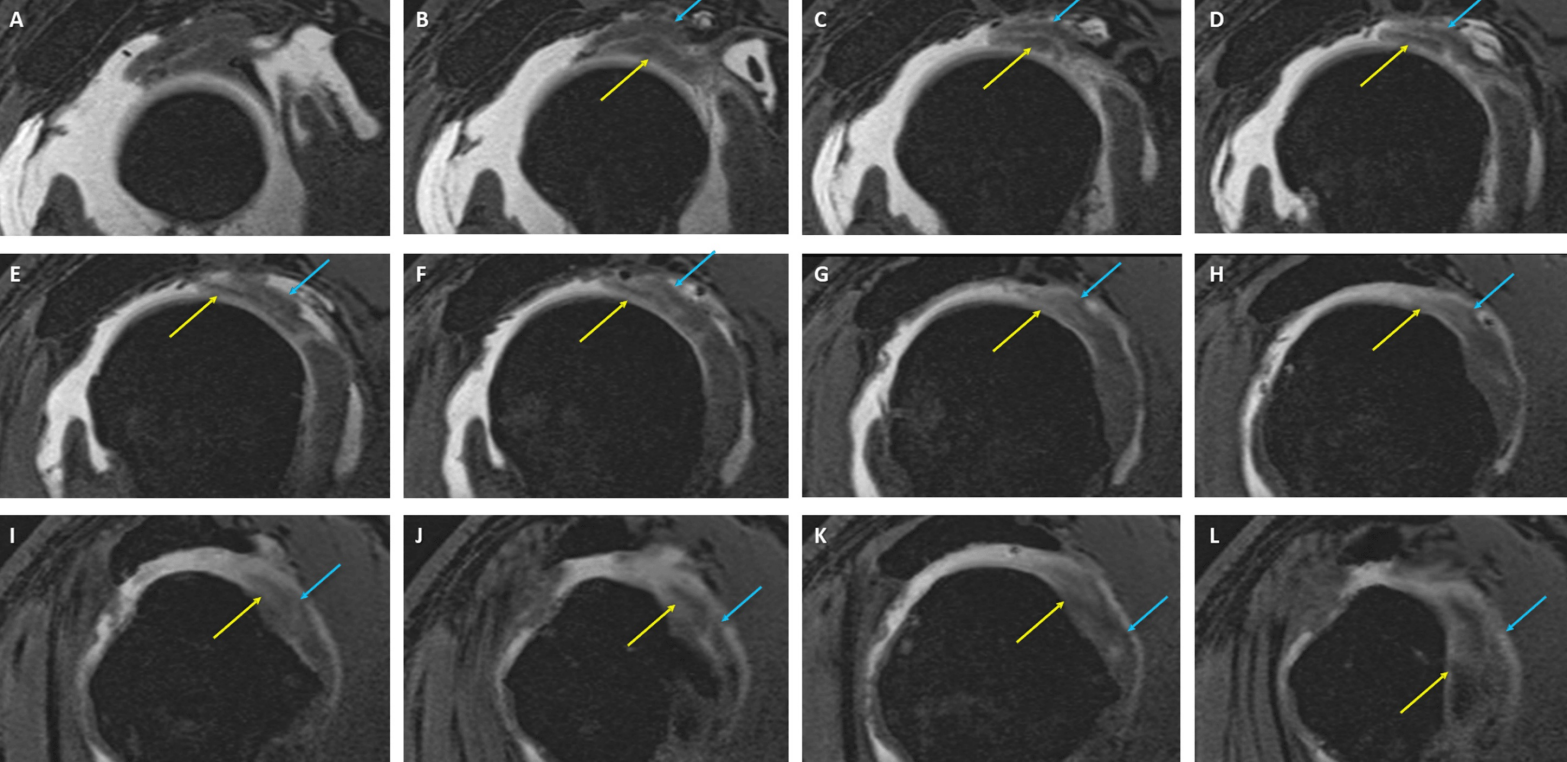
Sequential sagittal oblique T1-weighted VIBE MR arthrogram sections Consecutive sagittal oblique T1-weighted volumetric interpolated breath-hold examination (VIBE) MR arthrogram slices demonstrate nearly complete rupture of the supraspinatus and infraspinatus tendons and aponeurotic expansion of the supraspinatus tendon (blue arrows). Yellow arrows indicate the long head of the biceps brachii tendon.
